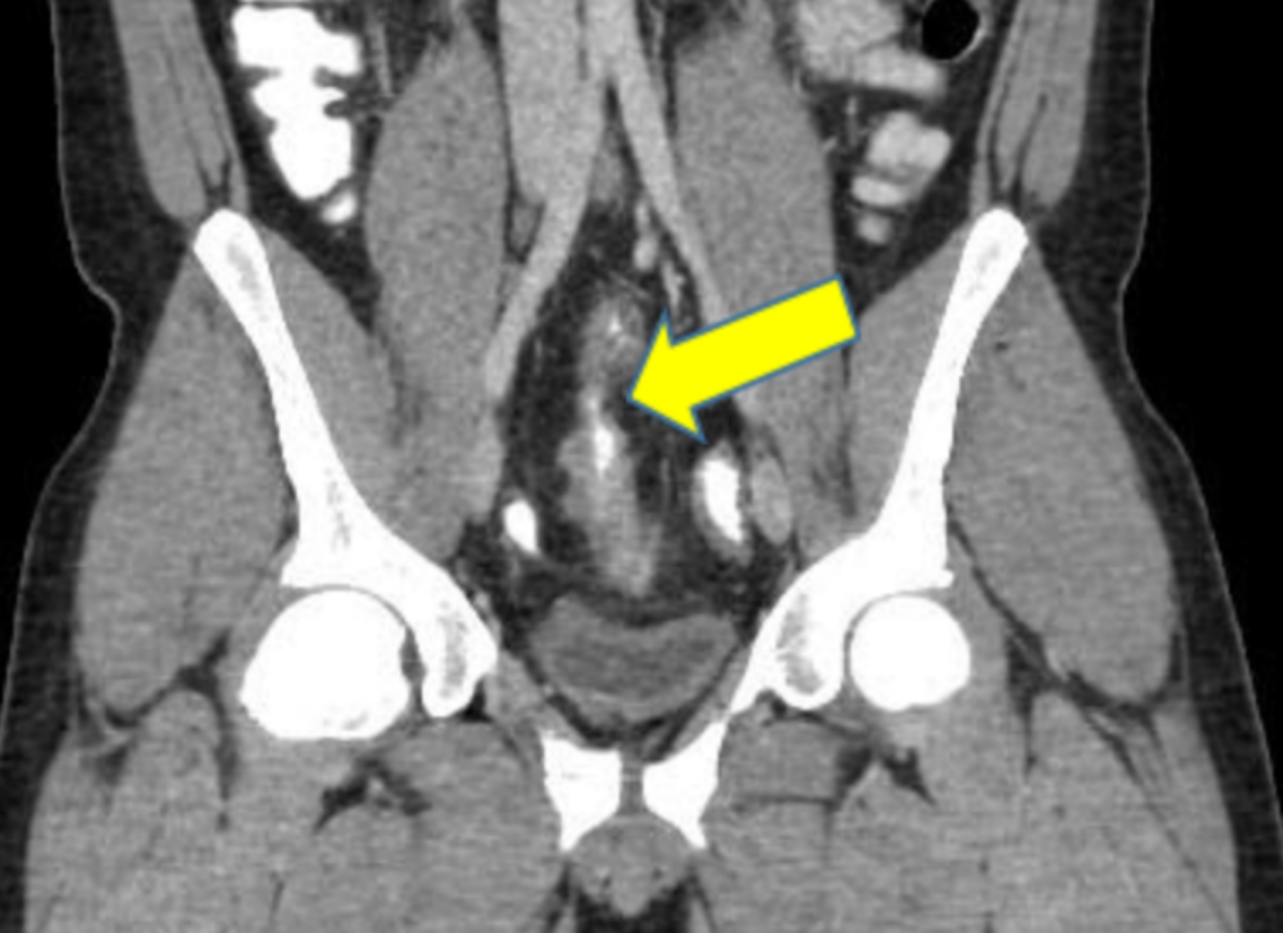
CT scan showing fistula between terminal ileum and sigmoid colon indicated by the yellow arrow
